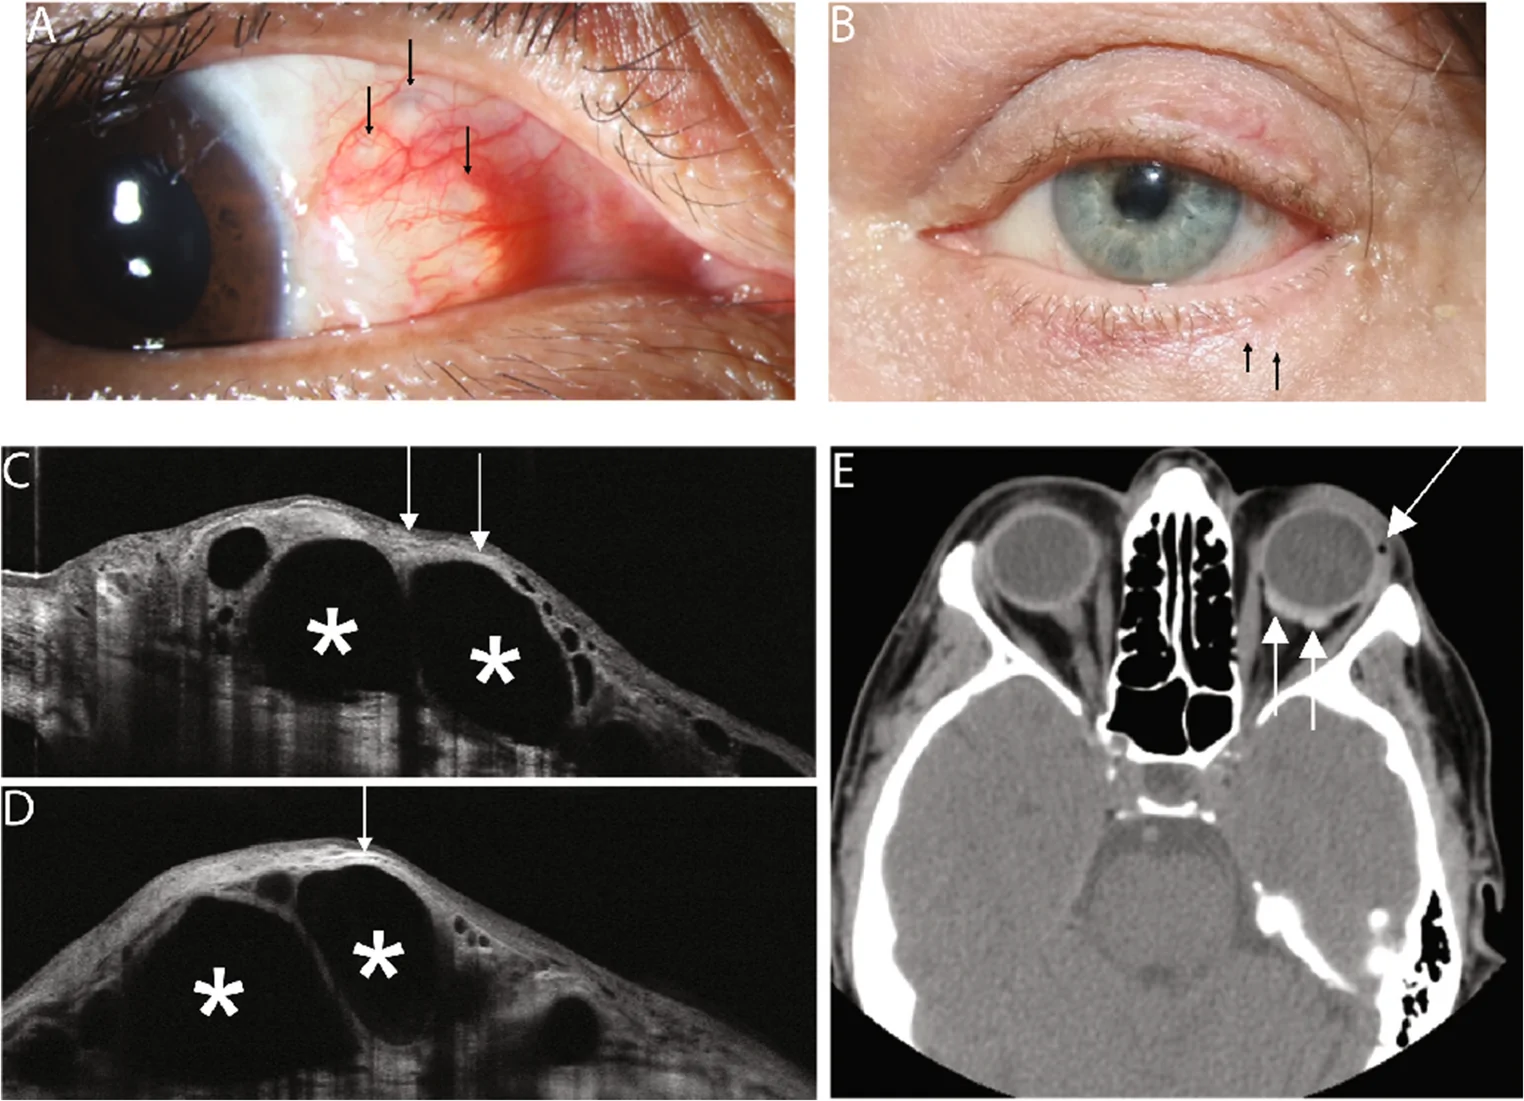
Representative images of silicone oil lesions in the bulbar conjunctiva and eyelid, and silicone oil lesions on anterior segment OCT and CT imaging of the brain and orbits. (a) Cystic lesion consisting of silicone oil present in the right nasal bulbar conjunctiva (arrows). (b) Extravasated silicone oil presents as a palpable lesion with fullness in the left lower eyelid (arrows). (c, d) Anterior segment OCT demonstrates an unremarkable hyporeflective epithelium with subjacent variably hyperreflective substantia propria (arrows). Underlying hyporeflective cystic spaces (asterisks) corresponding to silicone oil surrounded by histiocytes. (e) CT scan of the brain and orbits demonstrates thickening in a retrobulbar and lateral conjunctival location (arrows) corresponding to silicone oil migration with surrounding histiocytic infiltrate
